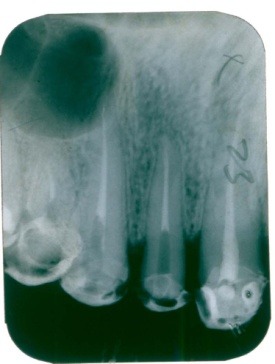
The restriction zone radiolucency - periapical and interdental bone recovery trend at 2.2
